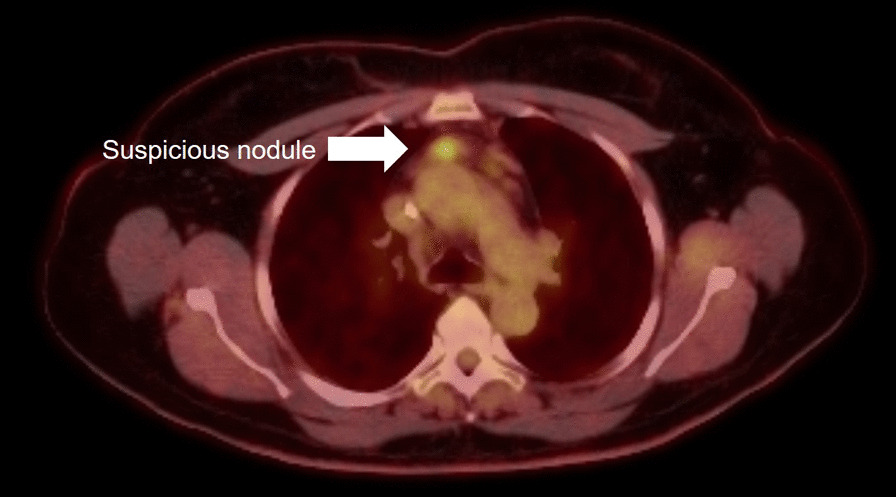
PET imaging of the anterior mediastinum identified a 2.2 cm prevascular lymph node with an SUV of 4.7
